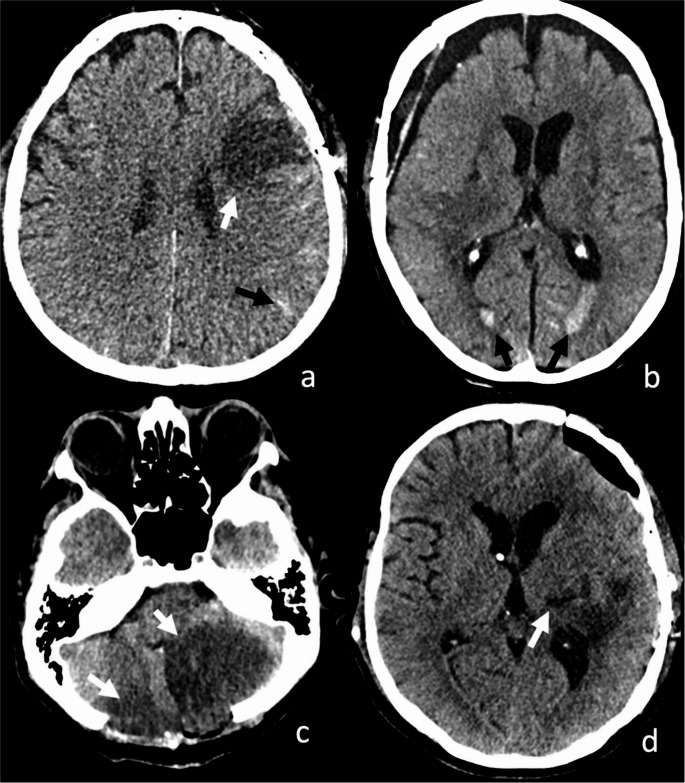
Examples of assessed dichotomous radiological variables. (a) Axial non-contrast CT showing a left frontal cortical infarct (white arrow) and subarachnoid hemorrhage along the left convexity (black arrow). (b) Axial CT demonstrating bilateral intraventricular hemorrhage within the occipital horns (black arrows). (c) Axial CT showing bilateral cerebellar infarcts (white arrows). (d) Axial CT demonstrating a left thalamic infarct (white arrow)
